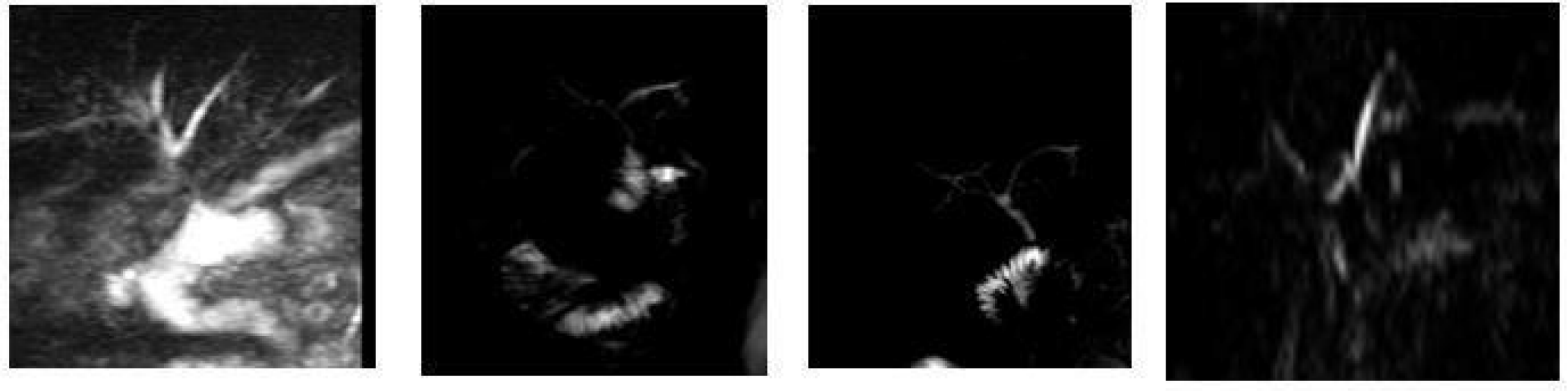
The latest magnetic resonance imaging in asymptomatic patients with elevated GGT levels.
